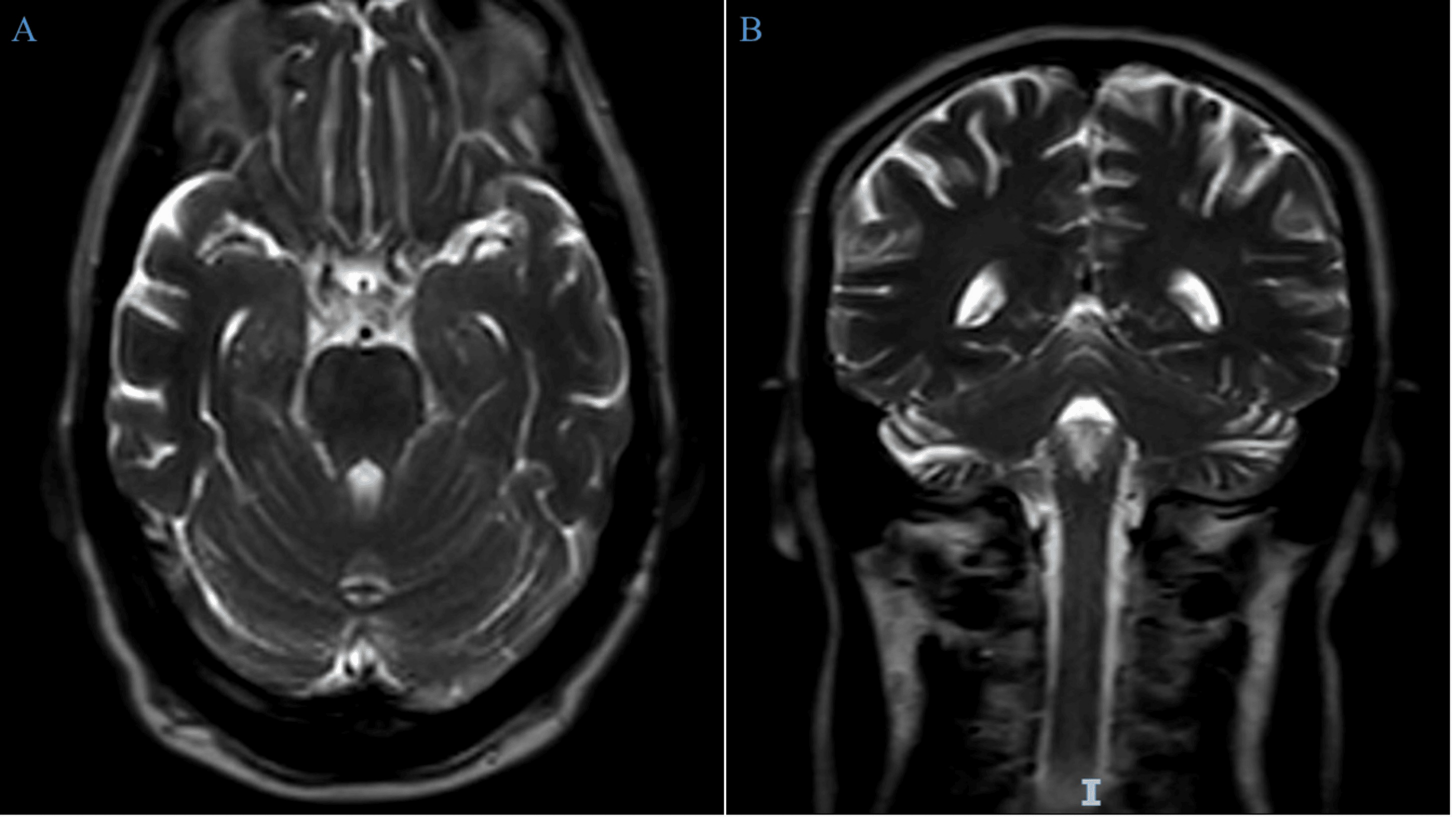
(A, B) MRI of the head with contrast MRI: magnetic resonance imaging
